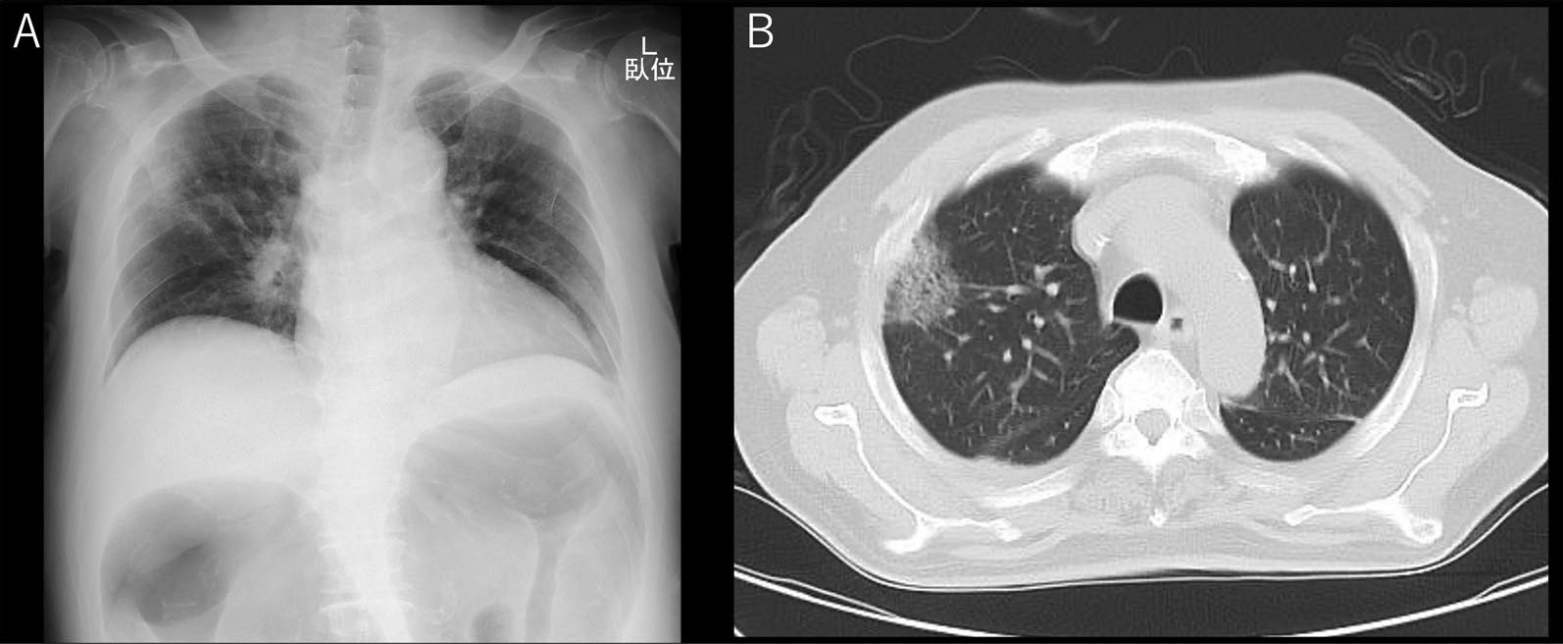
Chest radiography (A) and computed tomography (CT) (B) images obtained at admission: a chest radiograph showing opacities in the right upper lung field. Chest CT shows peripheral focal ground-glass opacity in the right upper lobes
